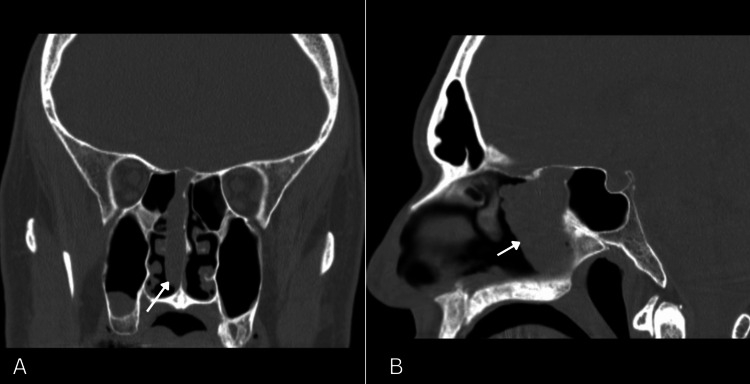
CT head imaging showing a polyp-like mass (white arrow) with indistinct separation from the middle turbinate, involving the skull base and extending inferiorly within the right nasal cavity. A. Coronal view CT head. B. Sagittal view CT head.
